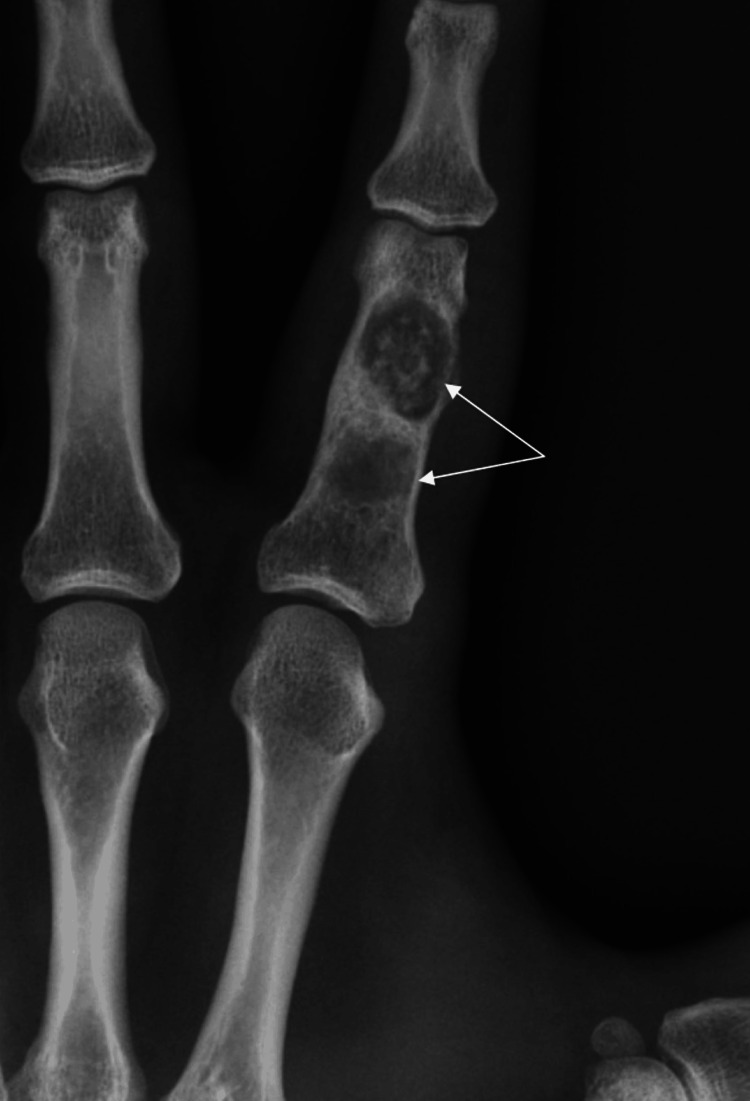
Preoperative X-rays (anteroposterior view) indicating the intraosseous lesions in the proximal phalanx of the index finger of the left hand (white arrows).
